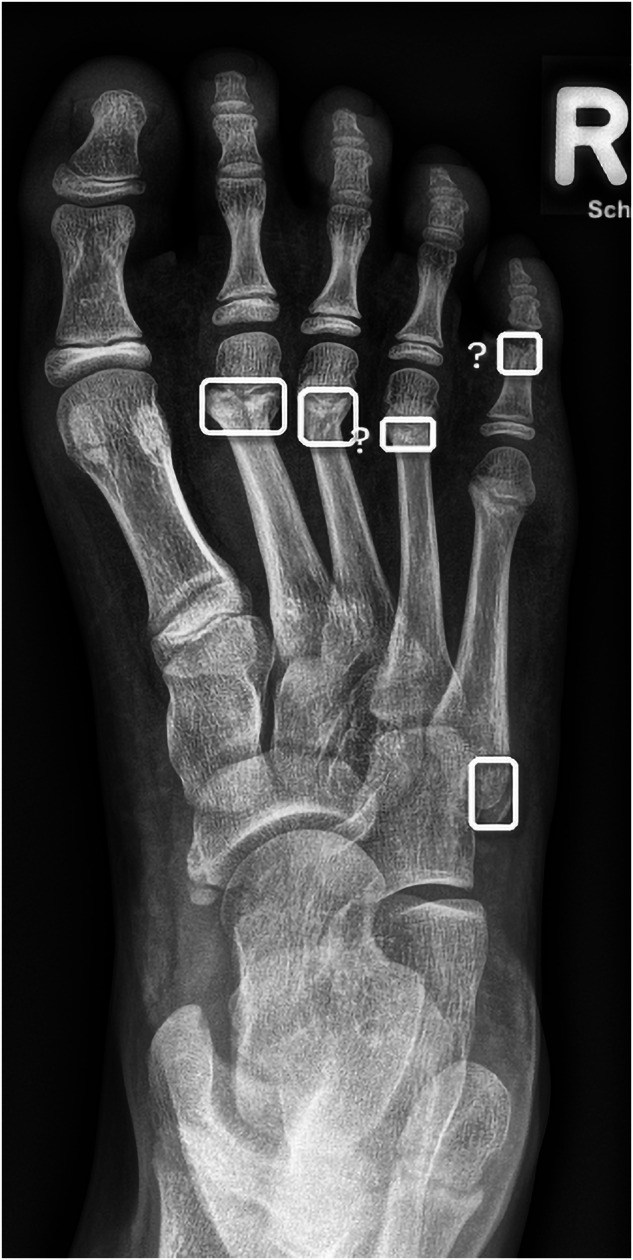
Radiograph of the right foot of an 8-year-old girl showing fractures of the distal second and third metatarsal bones. The AI incorrectly identified a false positive at the apophysis of the fifth metatarsal bone, as well as at the fourth metatarsal and proximal phalanx of the fifth toe. The latter two findings are marked with a question mark due to the AI’s low confidence level
